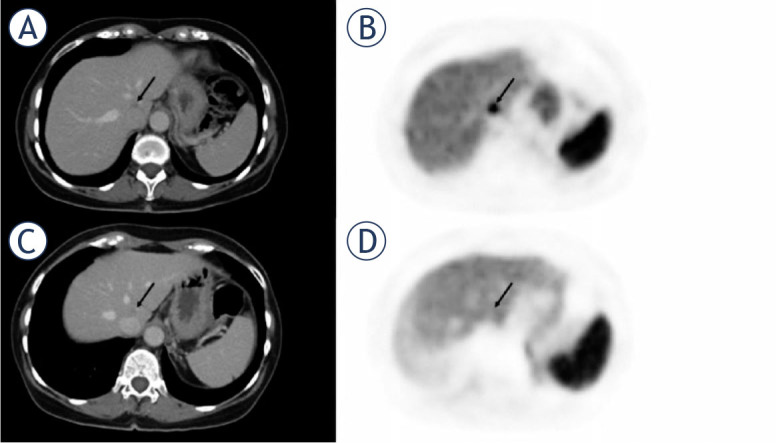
63-year-old female with responding liver metastasis of ileal neuroendocrine tumor. On the pretherapeutic PETCT (A, B) there were high tumor-to-liver (T/L) ratio. After three months of treatment with everolimus, the liver metastasis showed no significant shrinkage in size, but a significantly reduced uptake of ^68^Ga-DOTATATE (C, D) compared to pretherapeutic PET/CT (Tmax/Lmax: 0.94 *vs*. 2,09 and Tmean/Lmax 1.54 *vs*. 0.76).
